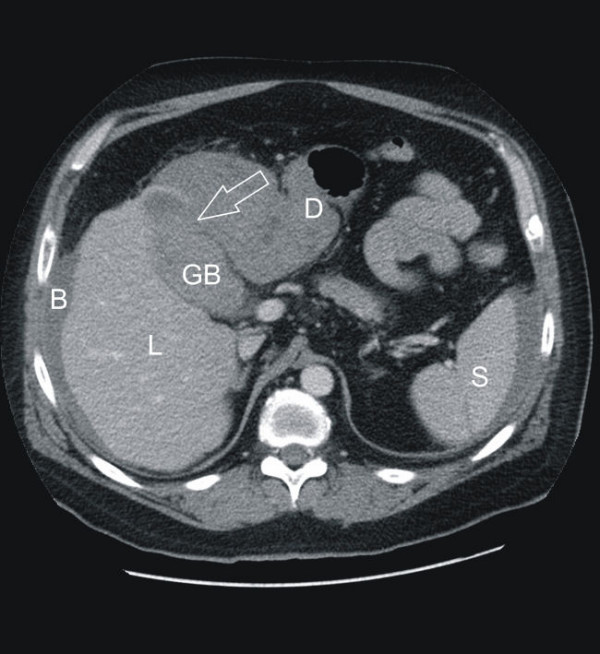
Computed tomography (CT) of the abdomen (a: axial slice). L = liver, GB = gallbladder, D = duodenum, S = spleen, B = blood. The perforation site is indicated by the transparent arrow.
